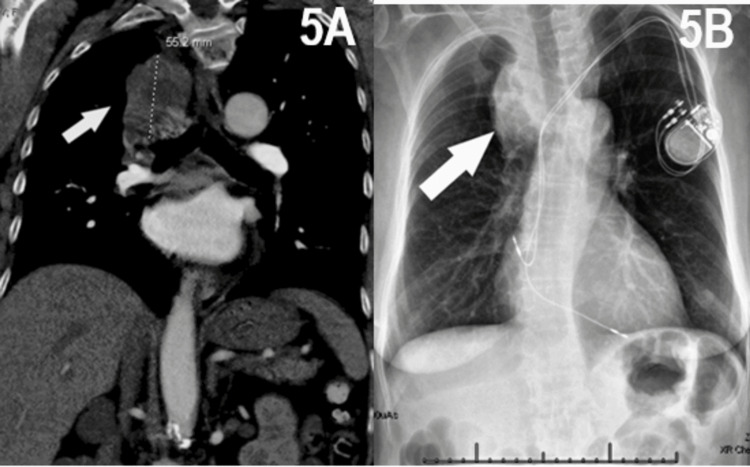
Chest CT (Figure 5A) Taken at the Time of Diagnosis and Chest X-ray (Figure 5B) Taken Two Months After the Initial Diagnosis, Both Demonstrating the Patient's Primary Right Upper Lung Mass 5A: Chest CT taken at the time of diagnosis prior to initiating the treatment identified a right upper lobe mass measuring 5.4*5.1 cm with central areas of necrosis, showing an interface with the trachea and esophagus and encasing the right upper lobe pulmonary artery with extension into the right main bronchus. This confirmed the suspicion of primary lung mass and was the initial diagnostic imaging. Follow-up imaging was conducted two months later. 5B: Chest X-ray taken two months after the initial identification of a right upper lung mass concerning malignancy. This imaging further progression of the previously identified mass with metastasis to paratracheal and 11 L and 4L thoracic lymph nodes, confirming stage IVC adenocarcinoma in this patient.
